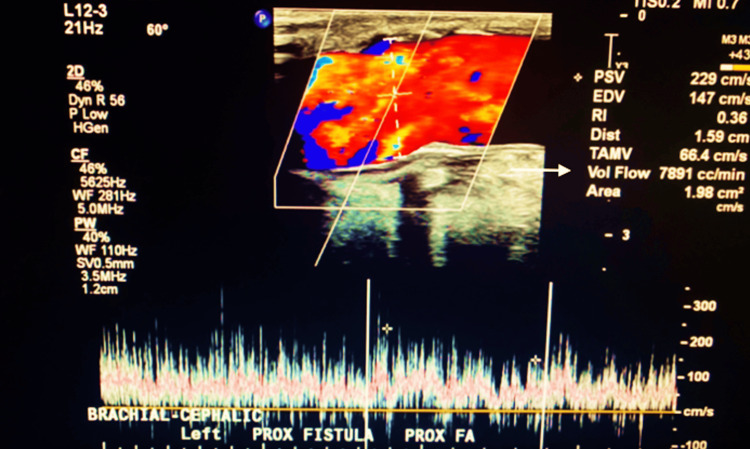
Preoperative duplex ultrasound consistent with a high-output AVF. AVF, arteriovenous fistulas
